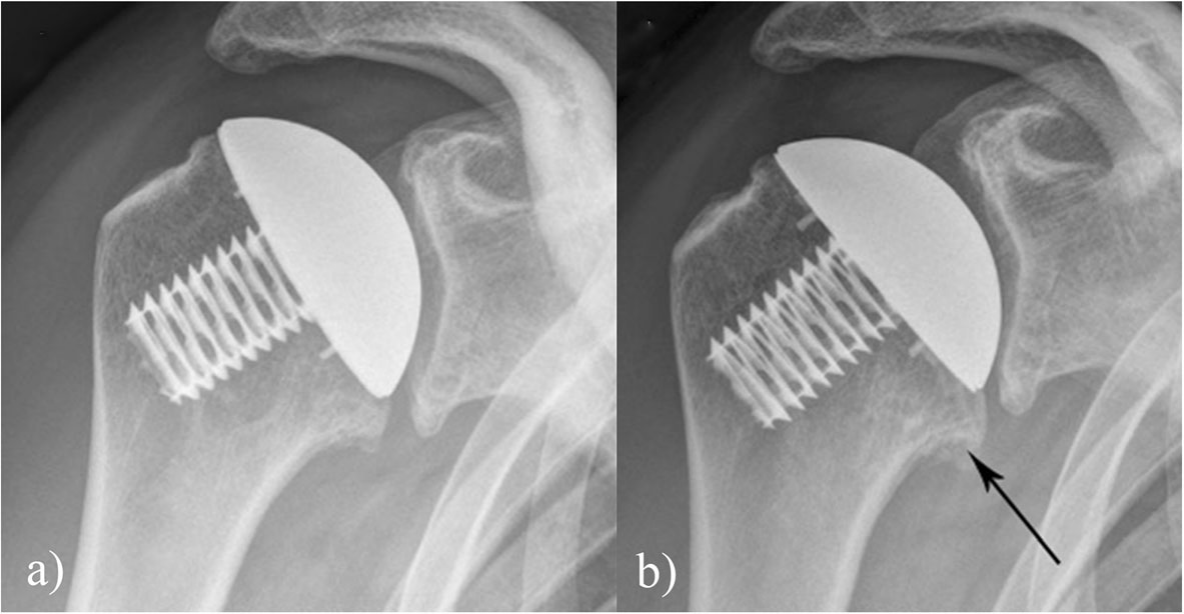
a Post operative image post surgery, b radiograph at eight years showing stress shielding around the calcar as indicated by the arrow
